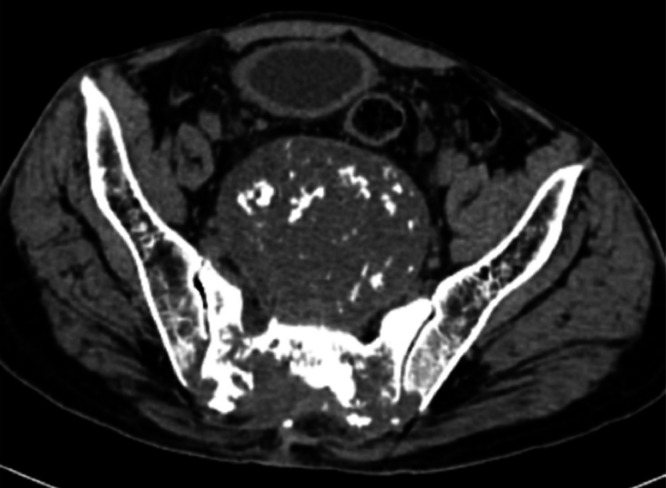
CT axial soft tissue window showing lesion involving presacral fat and displacing & compressing the rectum anteriorly and reaching upto subcutaneous region posteriorly, involving medial part of gluteal muscles.
